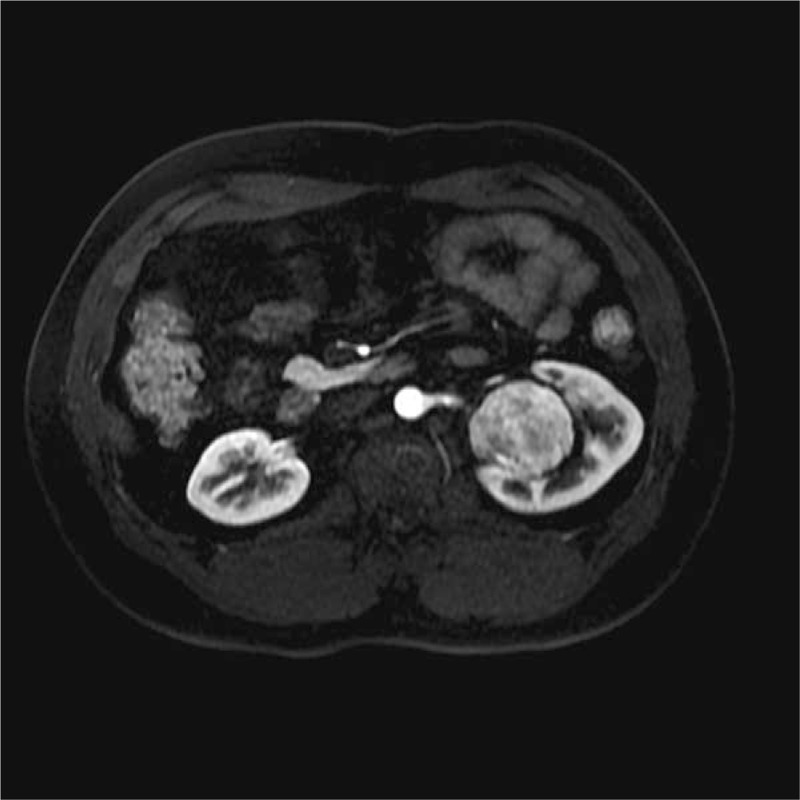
On dynamic contrast-enhanced magnetic resonance image, the arterial phase image showed unevenly mild enhancement.
